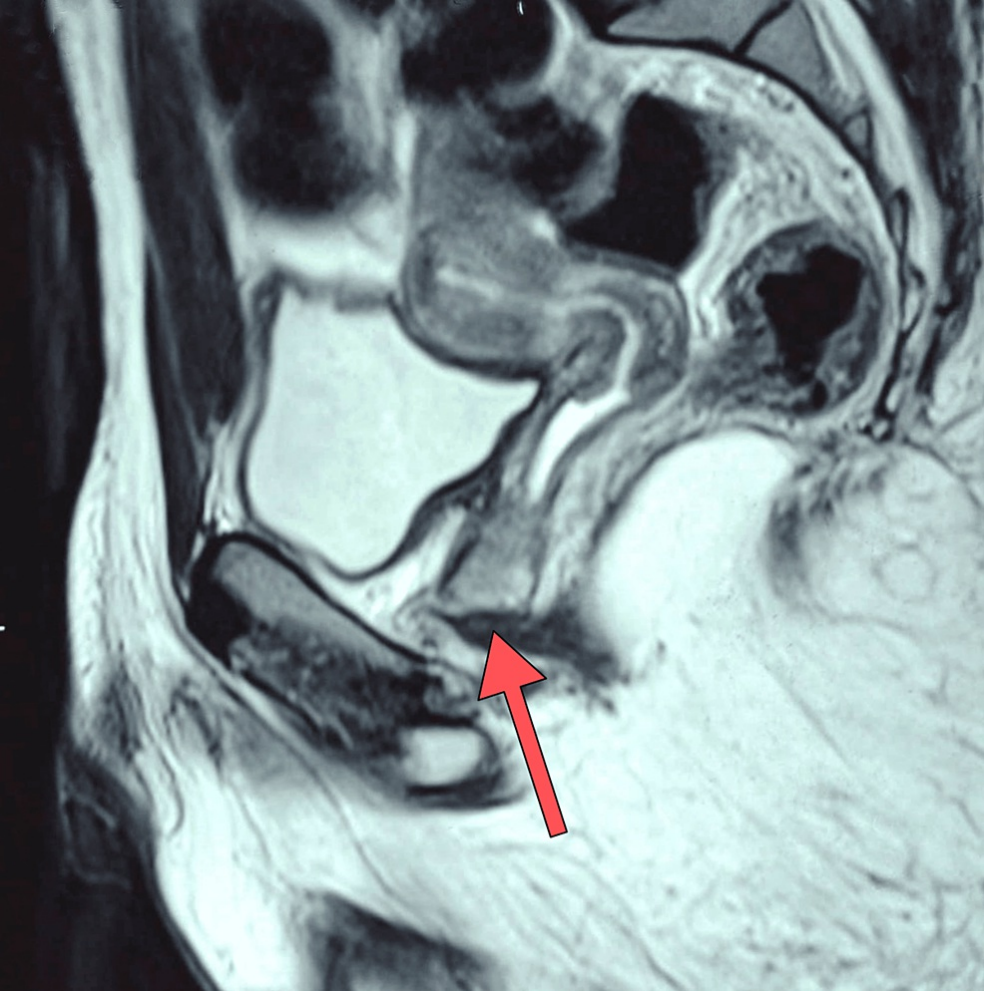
Magnetic resonance imaging of the pelvis. The uterus and vagina can be seen. Also, the abnormal connection between the vagina and posterior part of the urethra (red arrow) can be demonstrated.
